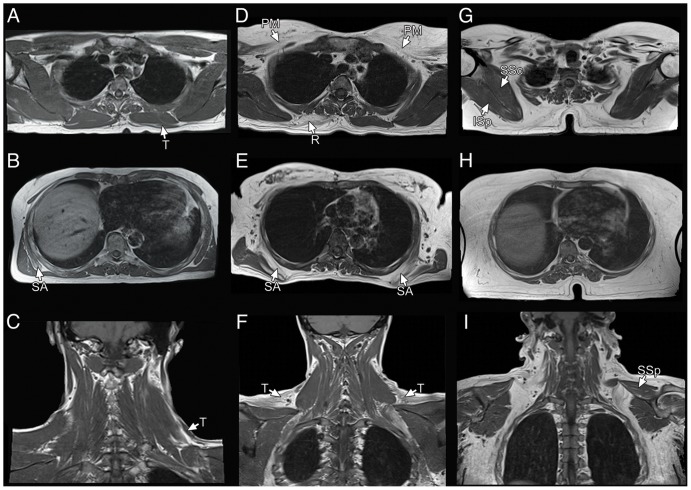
T1-W MR images in FSHD patients with different disease severity. In patients with initial disease (A–C) the involvement is generally restricted to the trapezius and serratus anterior muscles, often asymmetrically. In moderately affected patients (D–F), a frequent combination is constituted by bilateral involvement of the trapezius, serratus anterior, pectoralis major and asymmetric rhomboids involvement. In more advanced disease (G–I), other muscles become involved but still with typical complete sparing of the supraspinatus, infraspinatus and subscapularis. T: trapezius; SA: serratus anterior; PM: pectoralis major; R: rhomboids; SSp: supraspinatus; ISp: infraspinatus; SSc: subscapularis.
